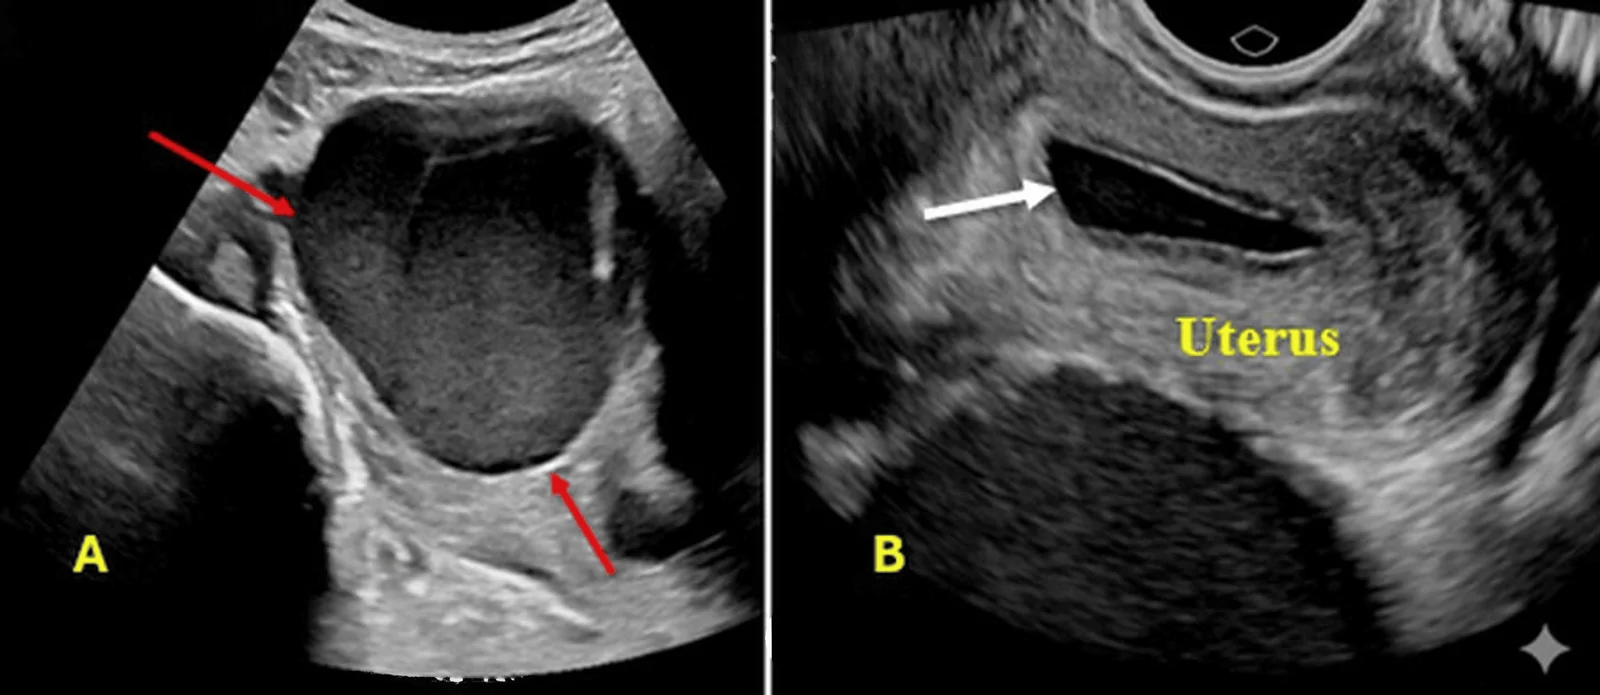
TVUS demonstrating hematosalpinx and endometrial fluid accumulation Red arrows indicate presumed severe hematosalpinx. Pyosalpinx is included in the differential diagnosis, as it may have a similar appearance. No evidence of ovarian torsion is identified. The white arrow shows a moderate increase in fluid within the endometrial canal. The cervix is not visualized. TVUS: transvaginal ultrasound
